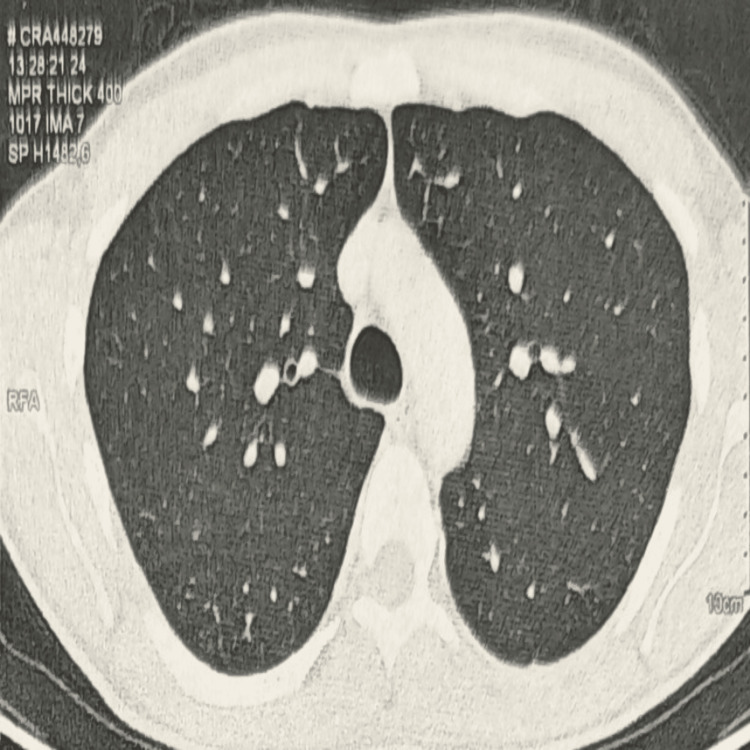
High-resolution axial thoracic CT demonstrates normal lung parenchyma bilaterally with no pleural effusion, mediastinal lymphadenopathy, or other abnormalities.
